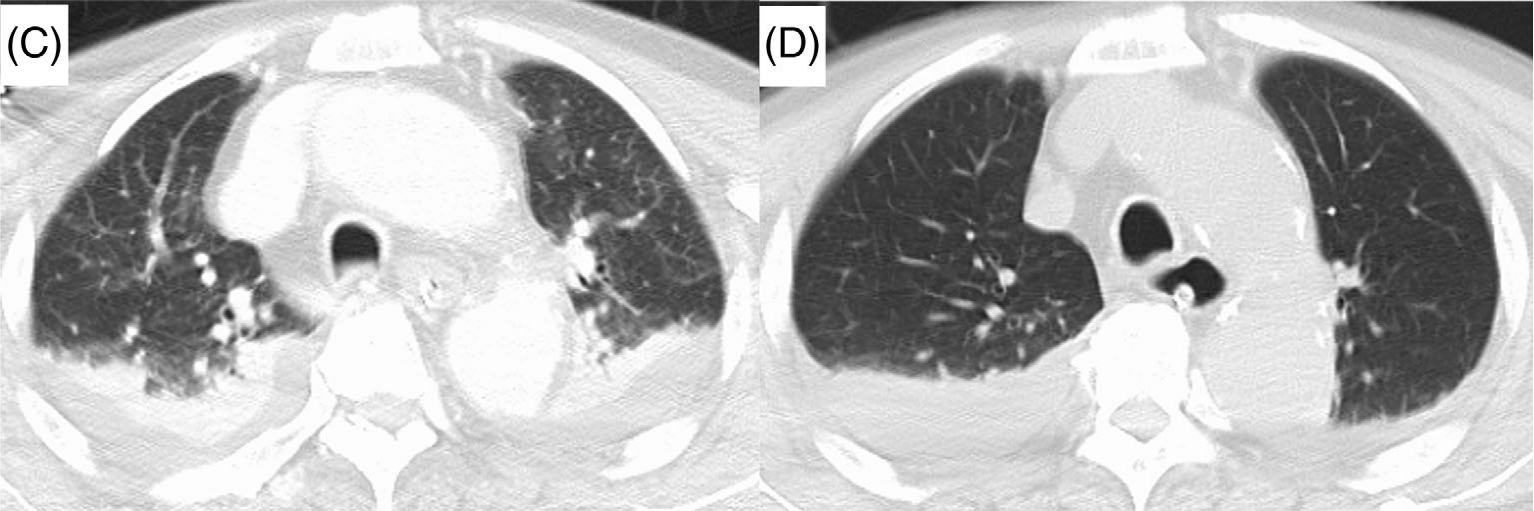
Chest computed tomography of case 2 before (C) and after (D) mechanical insufflation‐exsufflation
